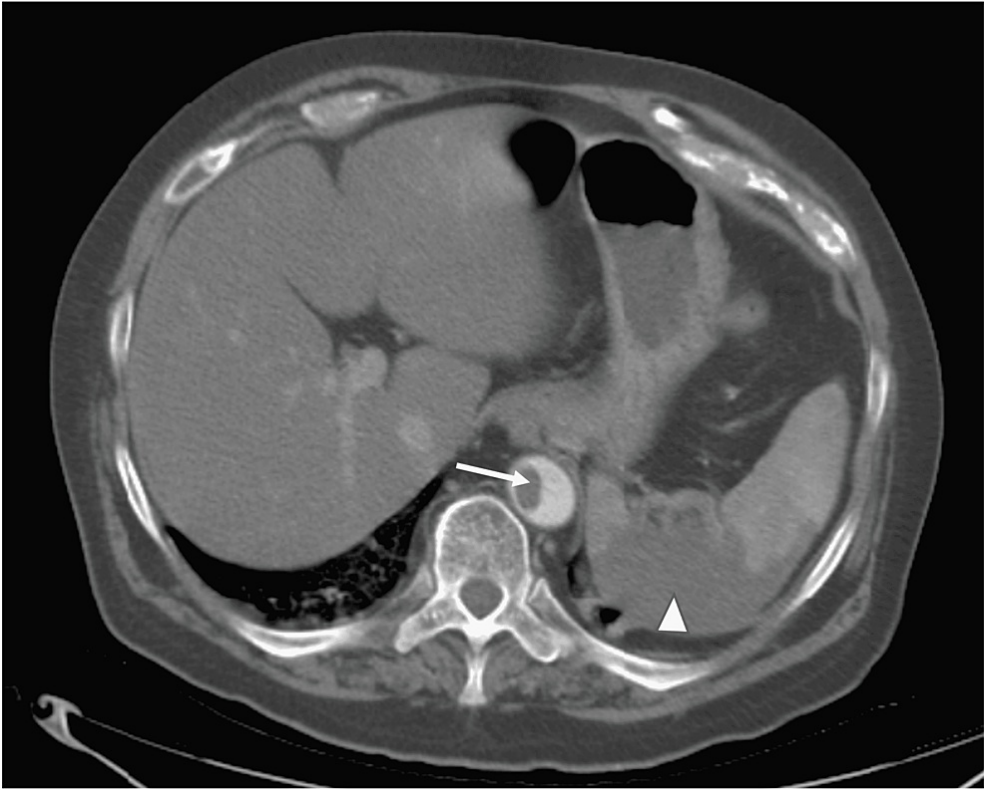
Computed tomography angiogram of the aorta showing thrombus within the splenic artery with wedge-shaped areas for possible infarction (arrowhead) and a 6 mm thrombus in the infrarenal abdominal aorta (arrow).
